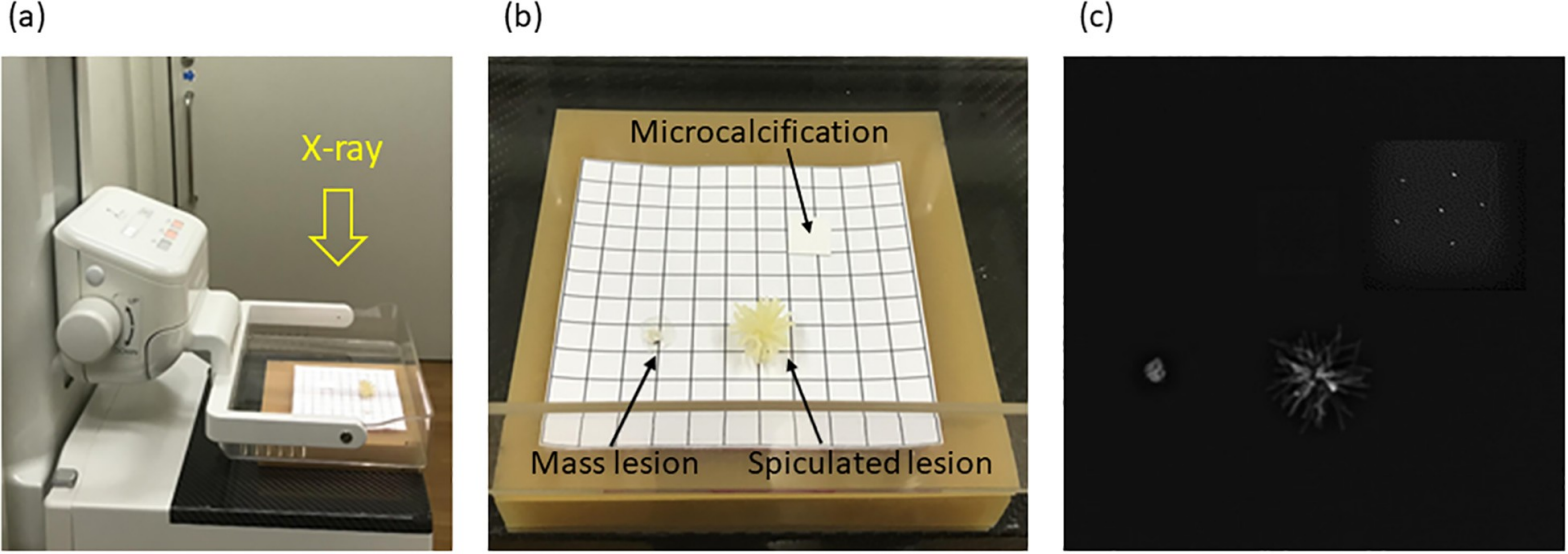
Preparation of receiver operating characteristic (ROC) practice. (a) Mammographic setting (b) An example of lesion placement (c) X-ray image of lesions corresponding to (b). Only microcalcifications are shown with magnification since actual size is only 100 μm in diameter.
